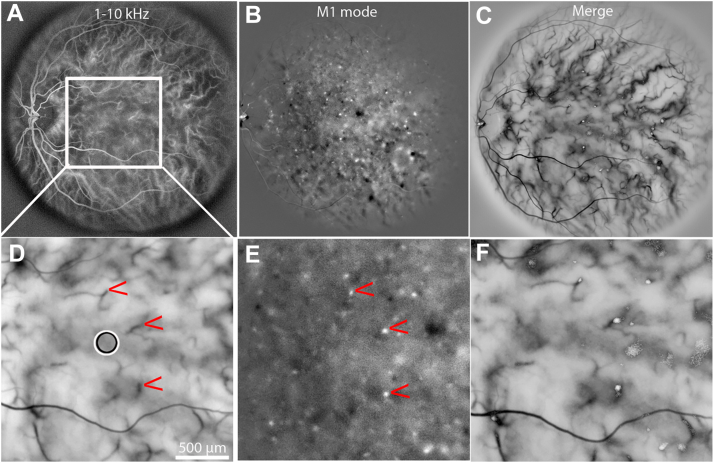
Organization of precapillary arterioles (same eye as in Figure 2). Panels A–C are magnified in D–F, respectively. The field of view in panels A–C is 40°. A, D, Laser Doppler holography image showing precapillary arterioles (examples are indicated by red arrowheads in D, which have been inverted to improve clarity; the circle indicates the fovea). B, M1 imaging showing anterograde axial flow. C, Merged images of A and B demonstrating that axial arterioles (in white) perfuse precapillary arterioles.
